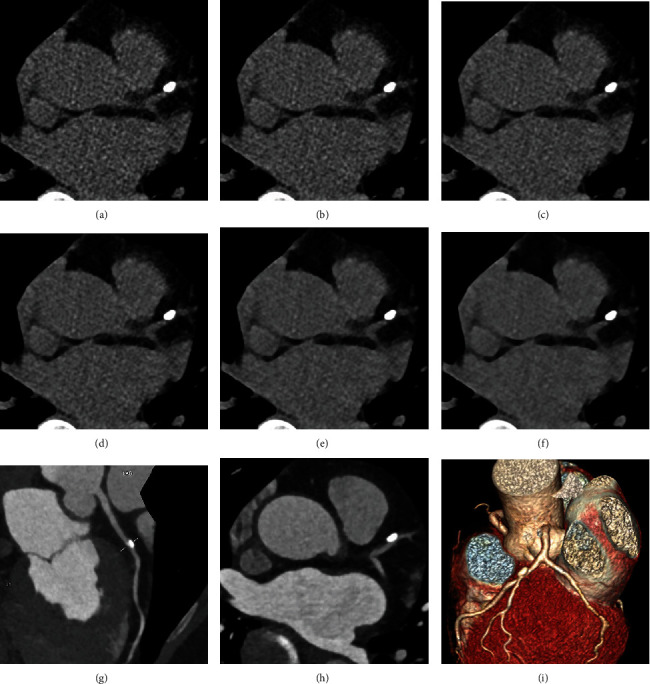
An example in a 61-year-old woman with suspected coronary artery disease. (a–f) Transverse CT images reconstructed with FBP (a), SAFIRE 1 (b), SAFIRE 2 (c), SAFIRE 3 (d), SAFIRE 4 (e), and SAFIRE 5 (f) and 3.0 mm reconstruction slice thickness. A calcified plaque located in the proximal segment of the left anterior descending artery is clearly shown in FBP and SAFIRE 1-5 images with the total Agatston score of 124.6, 104.3, 100.9, 92.6, 86.3, and 80.3, respectively. (g–i) Curved planar reformation of the left anterior descending artery (g), transverse CT image (h), and volume rendered reformatted image (i) in CCTA. A moderate stenosis caused by this calcified plaque is located in the proximal segment of the left anterior descending artery.
